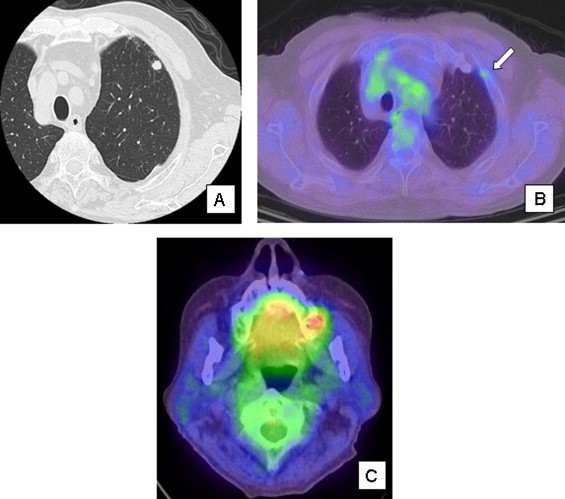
Chest CT on admission demonstrated the nodular lesion of 8 mm in diameter in the left upper lobe of lung. (A) Positron emission tomography demonstrated slight uptake of FDG at the position of the nodular shadow (white arrow) and no other abnormal findings were evident. Although FDG uptake of left cervical lymph node was increased, this uptake was supposed to nonspecific uptake due to nonspecific lymph node swelling (B) and (C).
